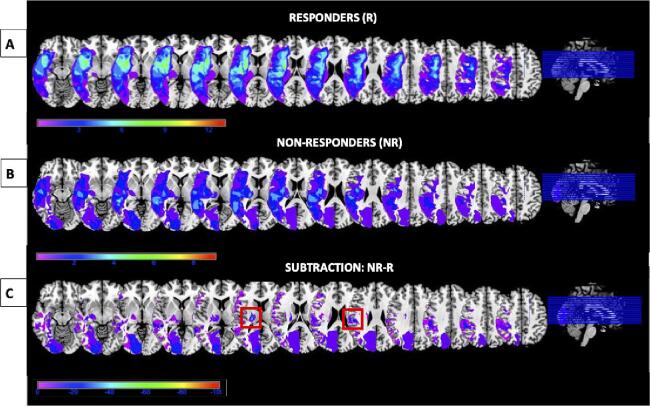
Neural correlates of tDCS response. Anatomical images (high-definition MPRAGE for 18 patients, clinical T1 MRI scans for 4 patients) were used for lesion analyses. Lesion maps were delineated on native space using the ImSeg Interactive Image Segmentation Tool (v1.8) developed by Dr Ben Glocker (Imperial College London). Binary lesion maps were normalised to a common stereotaxic space using the spatial normalisation routines of the Clinical Toolbox in SPM. Standard normalisation procedure and unified segmentation-normalization with enantiomorphic normalisation were employed for low-resolution images and high-quality images respectively. A. Lesion overlap for patients who performed better with real versus sham targeted tDCS (responders, n = 13). B. Lesion overlap for patients who performed worse or the same with real versus sham targeted tDCS (non-responders, n = 9). C. Lesion subtraction map showing the percentage of lesion overlap of non-responders after the subtraction of the overlap of responders. In light blue (inside the red squares), the right thalamus and the right postcentral gyrus which were associated with a lack of benefit from tDCS (MNI coordinates: X = 20, Y = -20, Z = 12 and X = 55, Y = –22, Z = 28 respectively). All resulting plots have been overlaid onto an MNI template brain. Colour bars reflect the relative frequency of damage. Note that although the frontal lobe may have been partially lesioned in patients, the area underneath the electrodes was spared as confirmed by a Consultant Neurologist who examined clinical scans prior to patient enrolment.
